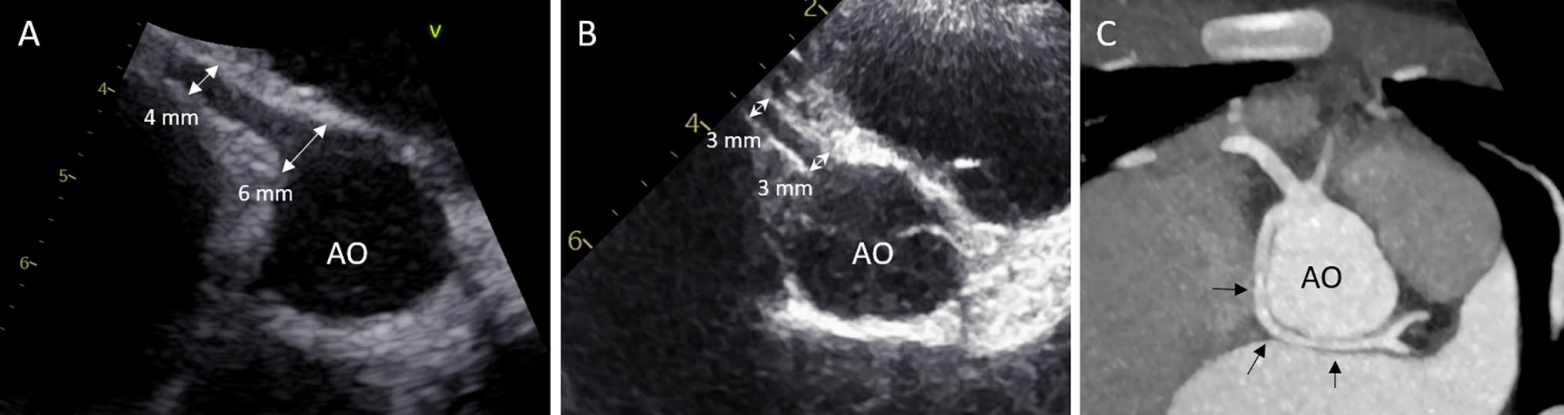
A transthoracic echocardiographic image at day 3: aortic root (AO) with a single coronary artery arising from the right aortic sinus; diameter 6 mm at ostium and 4 mm distally (white arrows). B transthoracic echocardiographic image at follow-up: diameters now decreased to 3 mm both at ostium and distally (white arrows). C computed tomography image: aortic root (AO) with a single coronary artery arising from the right aortic sinus and a left coronary trunk with retroaortic course (black arrows)
